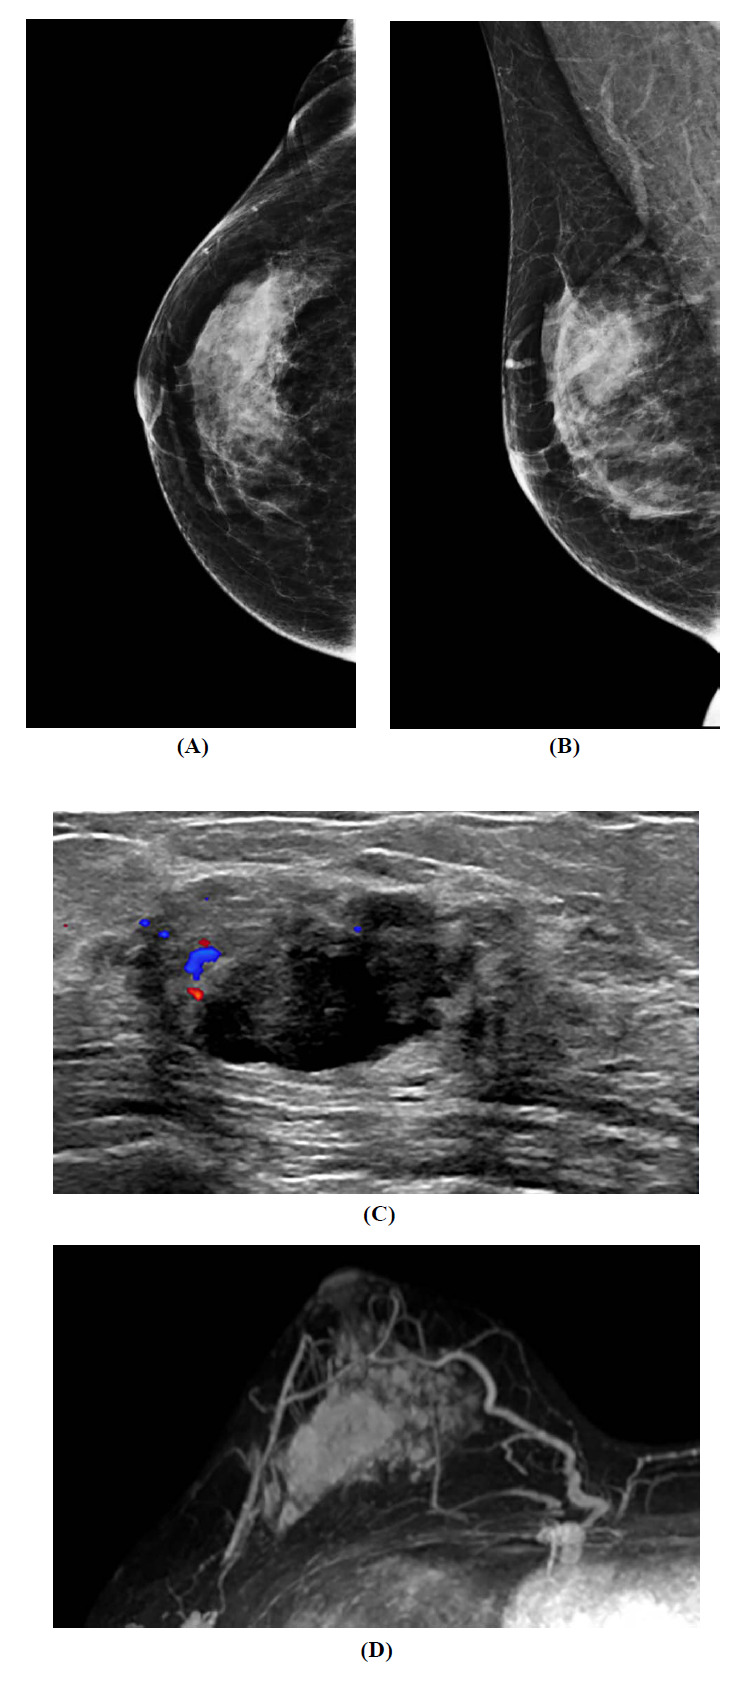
Findings of imaging study of the patient with initial invasive ductal carcinoma of the right breast. (A) Craniocaudal and (B) mediolateral oblique mammographic views reveal a 2.5 x 2.6 cm iso-dense, irregularly shaped mass with obscured margins located in the upper outer quadrant. (C) Ultrasound image shows a 3.2 x 1.5 x 2.8 cm sized irregularly shaped solid mass, with indistinct margins and increased vascularity on Doppler sonography. (D) Positivity for the adjacent vessel sign and prominent increased ipsilateral whole-breast vascularity in IDC-confirmed mass were observed on the maximum-intensity-projection image of the breast dynamic contrast-enhanced magnetic resonance imaging.
